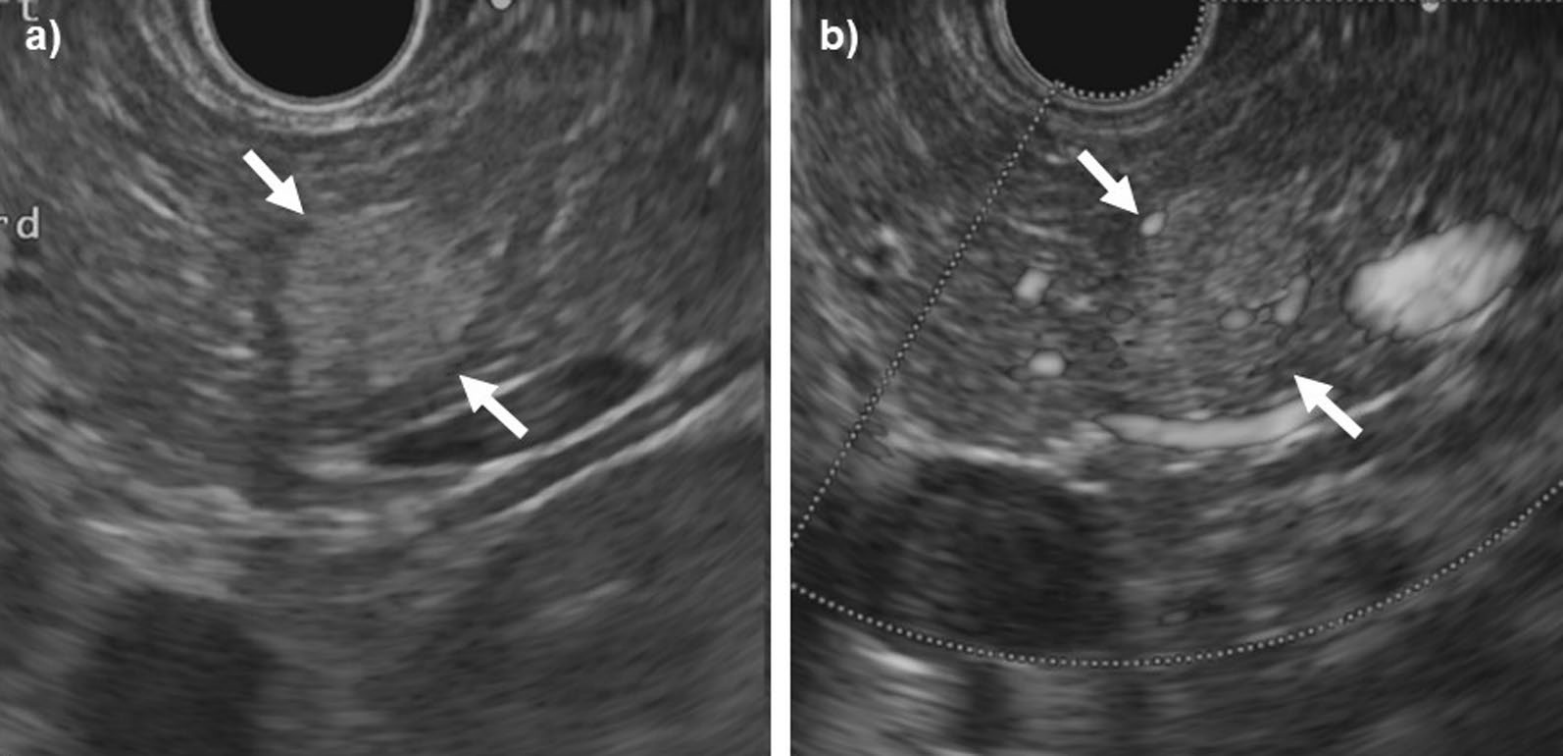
EUS. a B mode and b color Doppler. EUS revealed a hyperechoic mass with distinct borders and homogeneous interior in the tail of the pancreas (white arrows in a). Color flow Doppler imaging showed abundant internal blood flow inside the tumor (white arrows in b)
